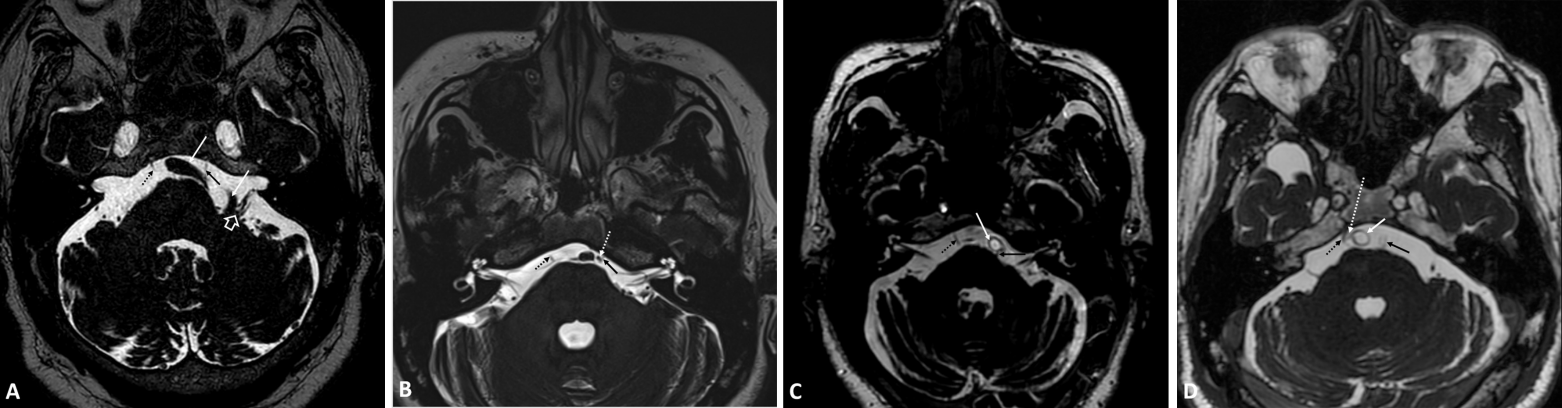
3D-FIESTA MRI sequence of cases 3 (A), 4 (B), 5 (C) and 6 (D).
